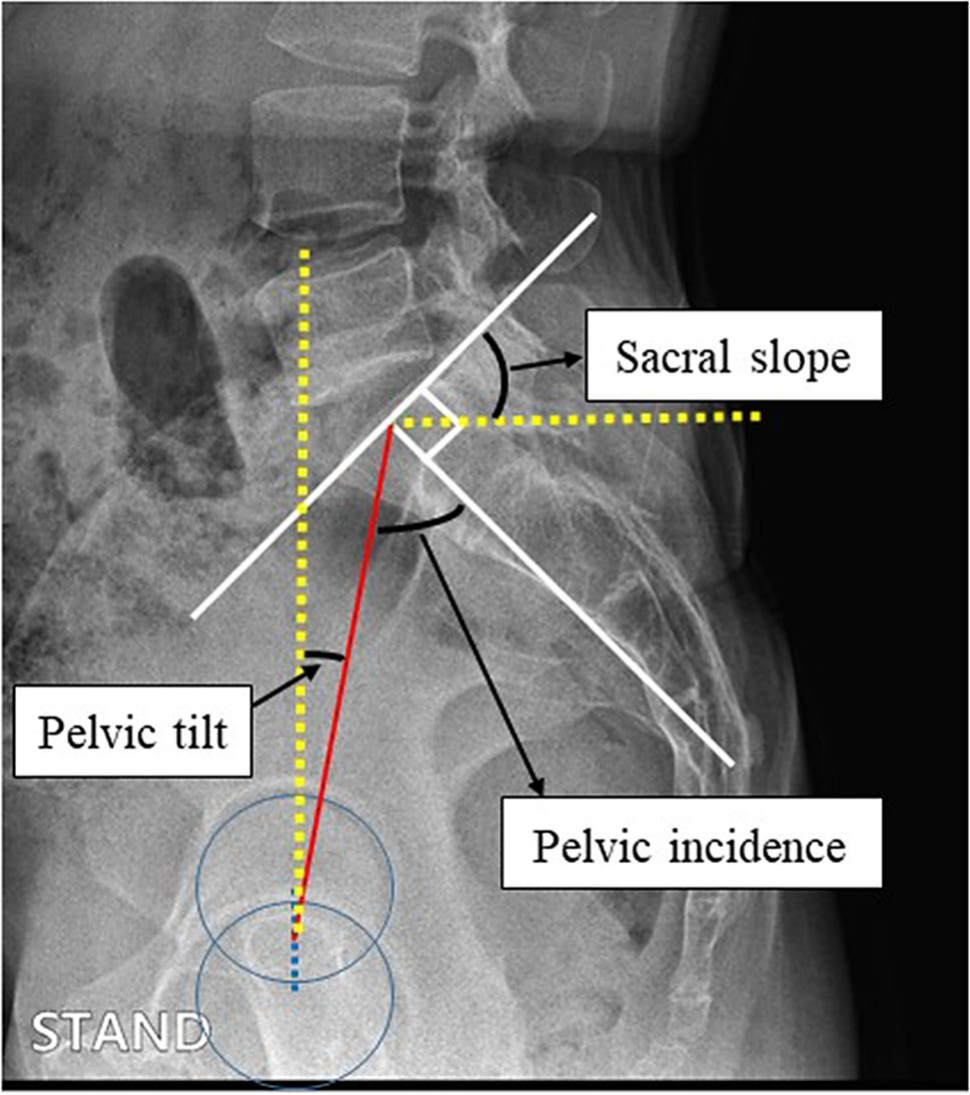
Pelvic sagittal parameters based on standing plain radiography
